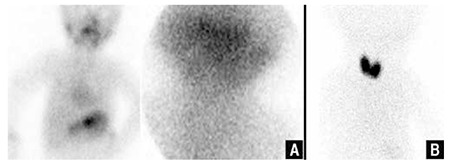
Thyroid scintigraphy revealing thyroid agenesis in a 6 month-old boy (A), increased Tc-99m O4 uptake in the enlarged thyroid gland in a one month-old boy with documented hydrogen peroxide generation defect (B)
